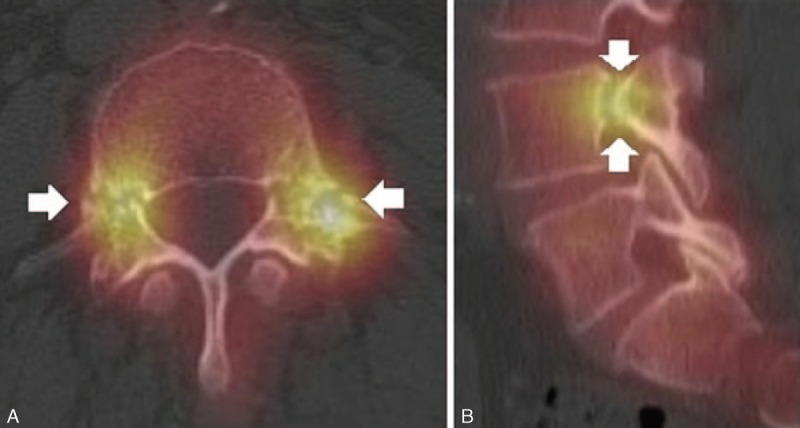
Hybrid SPECT–CT scan was conducted. (A) The SPECT-CT demonstrates the bilateral pedicle fracture through L4 (arrows). (B) The SPECT-CT parasagittal reconstruction image confirms L4 pedicle fractures (arrows). SPECT–CT = single-photon emission computed tomography–computed tomography.
